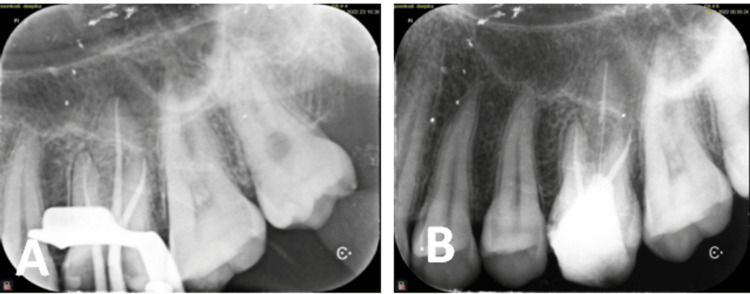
Intraoral periapical radiographs of 26 (A) Master cone verification and (B) postoperative radiograph
